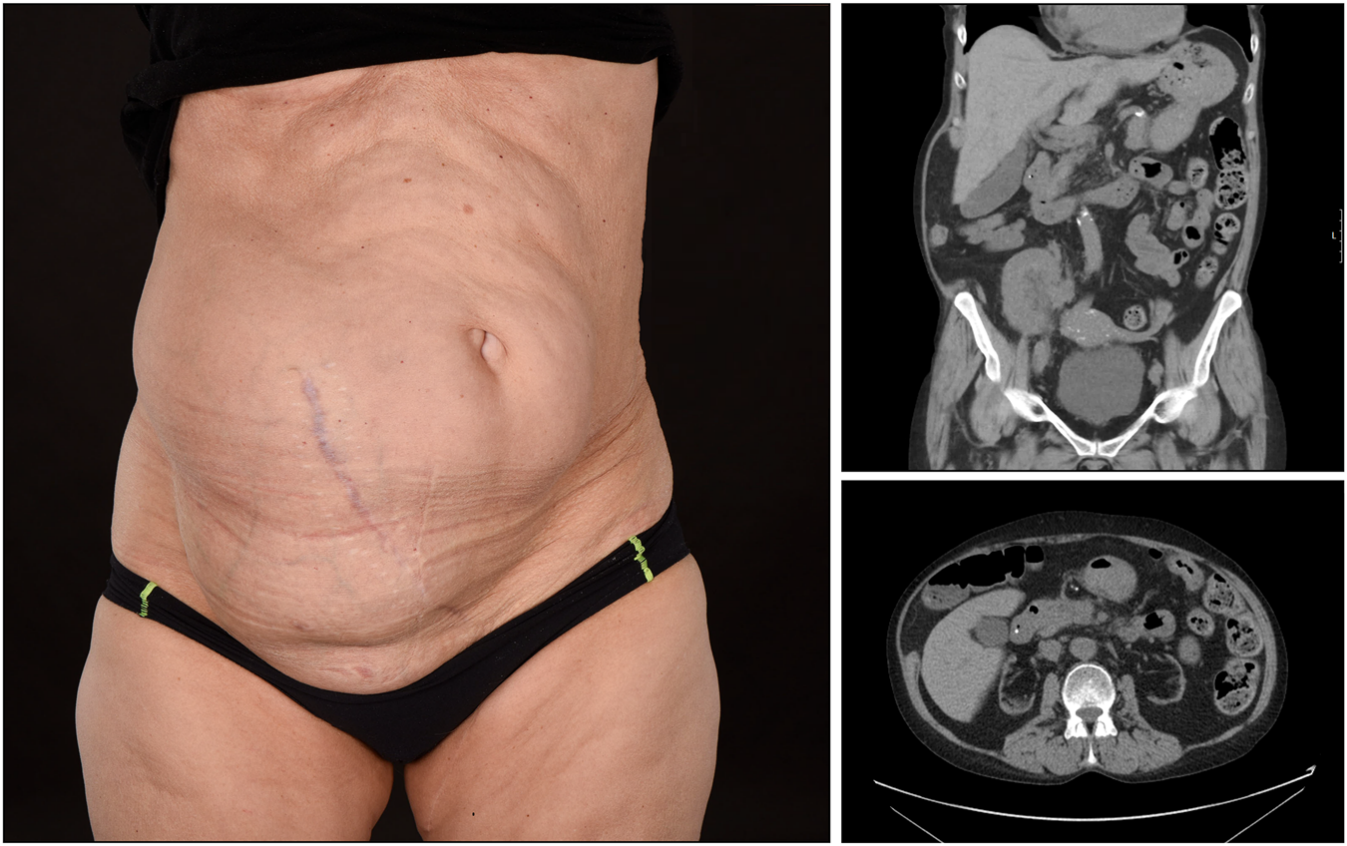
A study participant seeking care for right side protrusion of the lateral abdominal wall after kidney transplantation, the original CT report negating the presence of IH. Using the proposed classification, the IH is graded as a type 2B, 132 mm. The IH was later intraoperatively verified during hernioplasty. The study participant has consented to the use of these images
